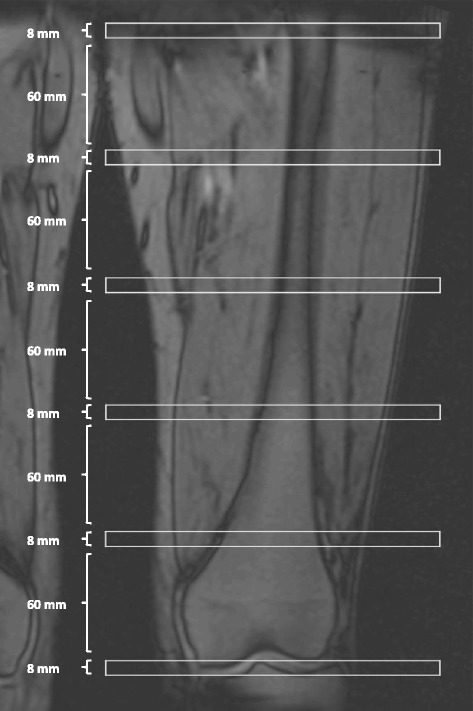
Magnetic resonance imaging analysis. We place slices as shown for analysis of cross-sectional area of the m. quadriceps femoris muscle and analyze slices 3 (counting in distal to proximal direction) and 4 for all subjects, and we use slice 4 for primary outcome evaluation. We fix the placement of slices in absolute distances, but we measure the femur length on dual-energy X-ray absorptiometric scans from the lateral tibial plateau (0 %) to the top of the greater trochanter (100 %) to report the relative placement of slices. Currently, placement of slice 3 ranges from 27 % to 36 % and slice 4 from 40 % to 54 % of the femoral length, depending on the height of the participant
